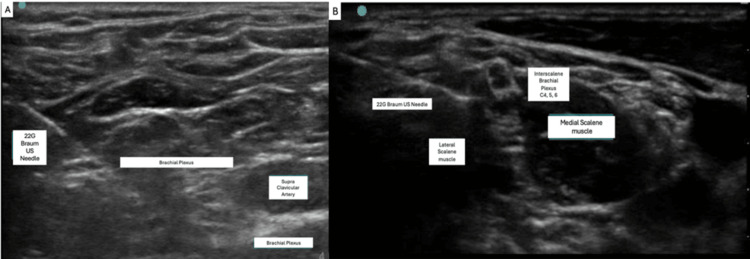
Ultrasound images of supraclavicular and intrascalene level. Ultrasound images of the supraclavicular (A) and the interscalene level (B), which were used to guide ropivacaine injection during the case.
